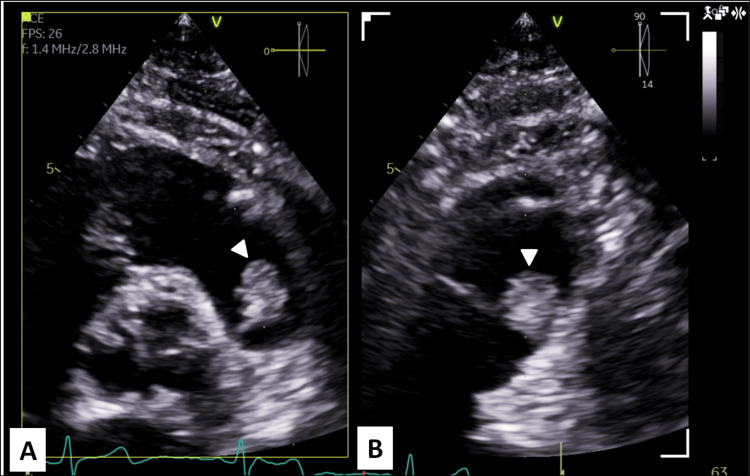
Parasternal Short-Axis Transthoracic Echocardiography (A) and (B) demonstre transthoracic echocardiographic parasternal short-axis views with multiplanar imaging, revealing a mobile, heterogeneous mass attached to the pulmonary valve (arrowheads).
